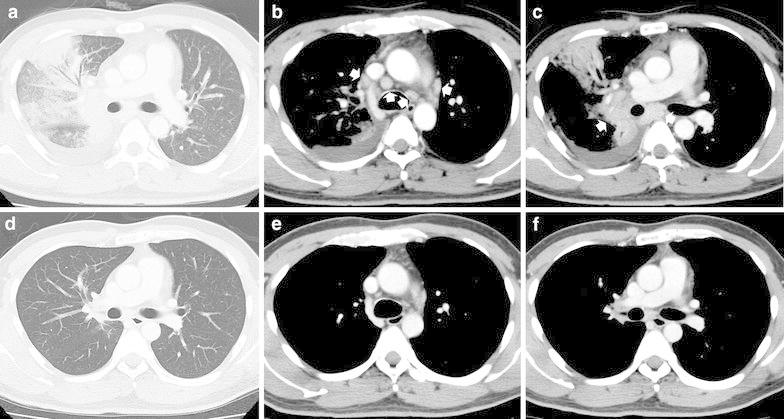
Chest enhanced CT images (a, d lung parenchyma window; b, c, e, f mediastinal window). a–c Diffuse air-space consolidation in the right lung with ipsilateral pleural effusion and massive mediastinal and hilar lymphadenopathy (*arrows*) at admission. d–f Disappearance of consolidation, pleural effusion and lymphadenopathy after the treatment
